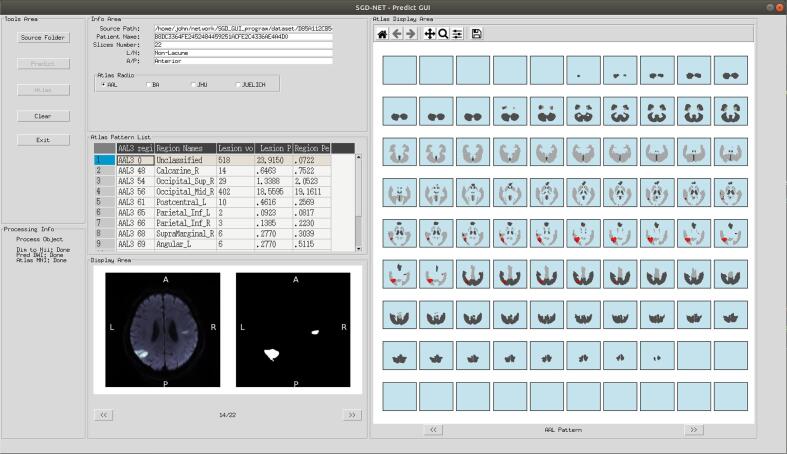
SGD-Net and SGD-Net Plus for AIS lesion report. The user interface provided the results of SGD-Net classification for AIS lesion size (lacune and non-lacune) and lesion location (anterior and posterior circulatory territory) and the results of SGD-Net Plus for AIS lesion volume in each brain region, lesion percentage in that brain region, and region percentage occupied by the lesion. It also visualized the original DWI images, S1 segmentation, and serial cuts for the atlas with red-marked AIS lesions. (For interpretation of the references to colour in this figure legend, the reader is referred to the web version of this article.)
